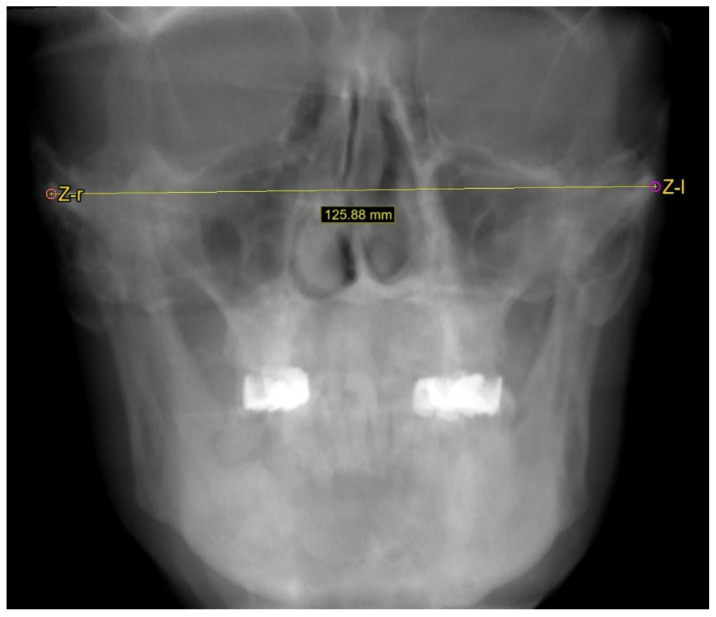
Frontal cephalometric radiograph. Z-r, Z-l—zygion points (right and left). The facial width was measured.
